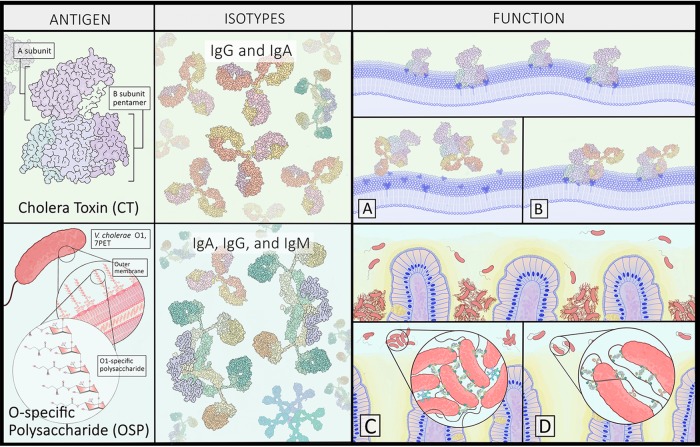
Cholera toxin (CT) and the O-specific polysaccharide (OSP) are the two dominant V. cholerae antigens. Remarkably, following infection in an area of endemicity, more than 75% of the antibodies derived from clonally expanded plasmablasts targeted either CT or the OSP. CT antibodies target both the A and B subunits and may block toxin binding (A) or activity (B), yet the persistence of circulating antitoxin antibodies does not appear to confer long lasting immunity to cholera. OSP-specific antibodies target the bacterial outer membrane and confer protection. Several mechanisms have been proposed, including agglutination (C) and motility inhibition (D) or other effector functions which may entrap V. cholerae before it reaches the mucosal surface, such as activation of neutrophil extracellular traps.
